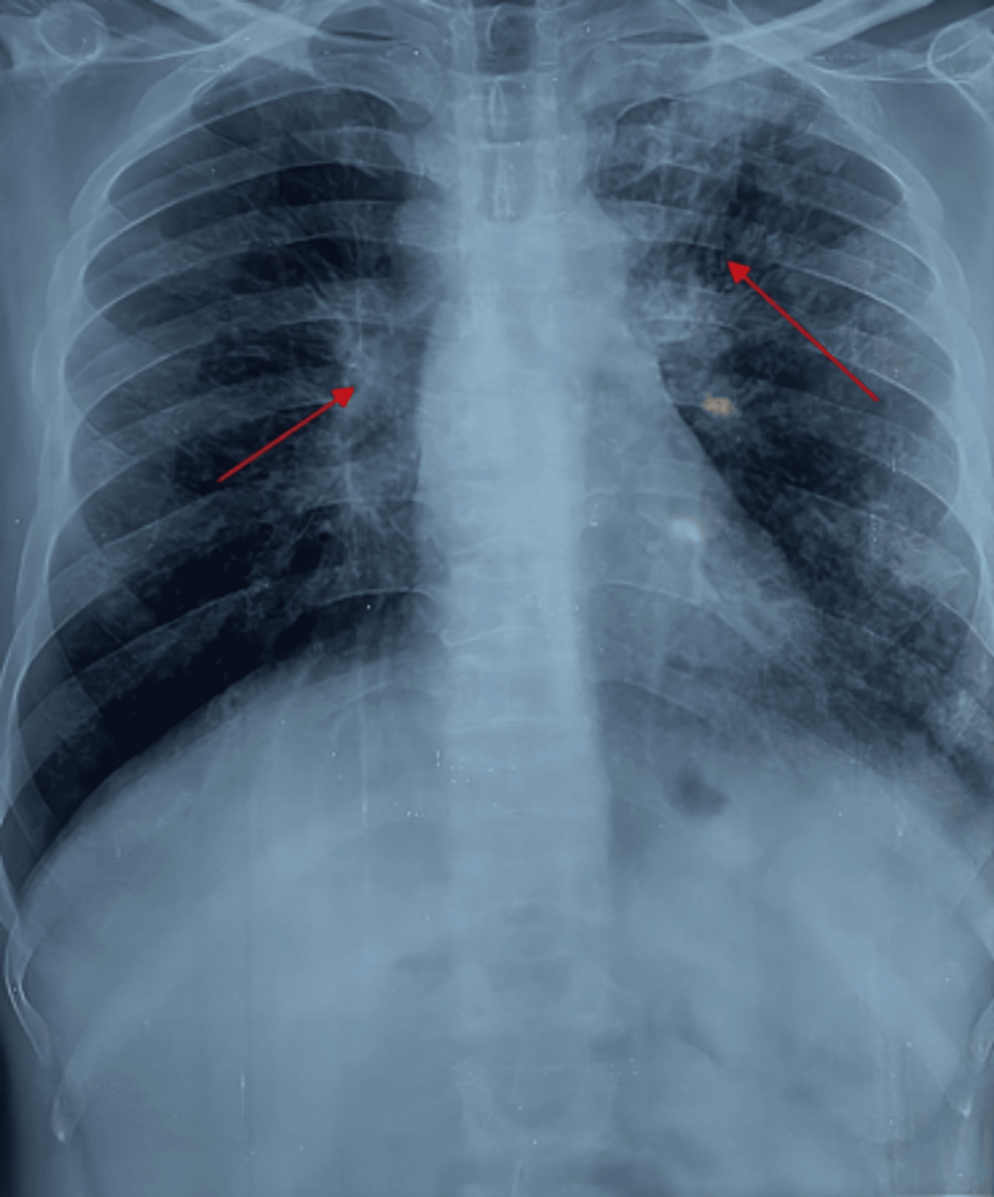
Chest X-ray-postero-anterior (PA) view showing bilateral diffuse nodular opacities
